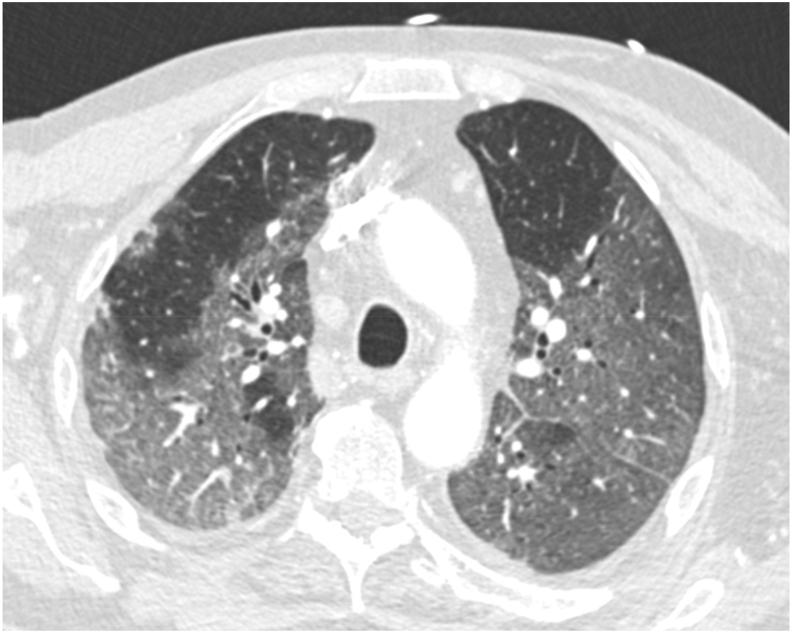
A chest CT showing bilateral ground-glass opacities suggestive of COVID-19.
